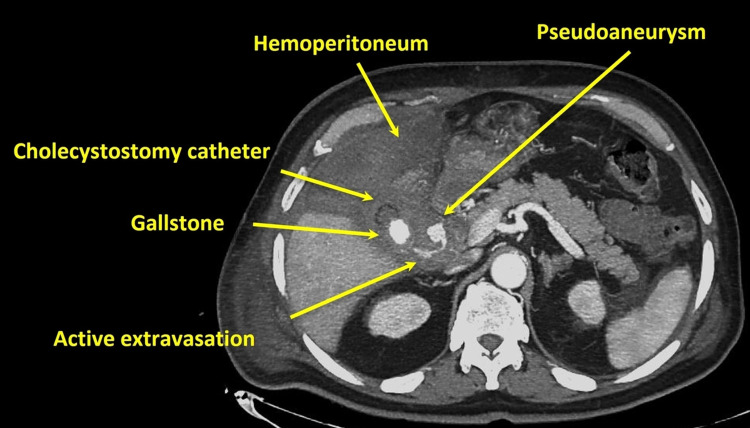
Axial CT angiography reconstruction indicates active extravasation from cystic artery pseudoaneurysm and hemoperitoneum in perihepatic space.
